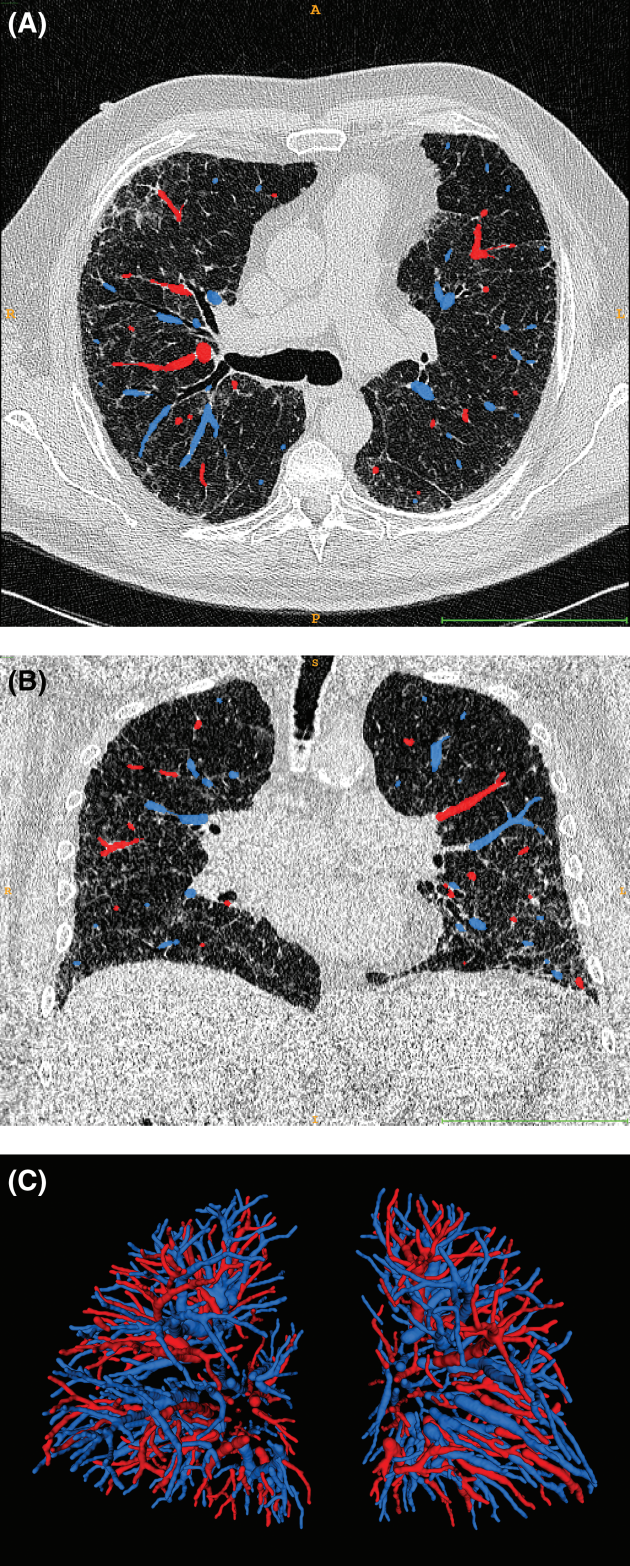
Transversal (A) and coronal (B) thoracic computed tomography (CT) images of a representative patient with overlays of the arteries (blue) and veins (red) and 3D rendering of the arterial and venous vessel trees in this patient (C). The bar at the bottom of (A) and (B) is 10 cm wide.
